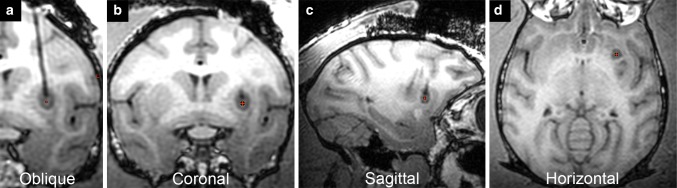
Real-time intraoperative MRI for intracerebral delivery of stem cells provides feedback of cannula placement and infusion site: a
